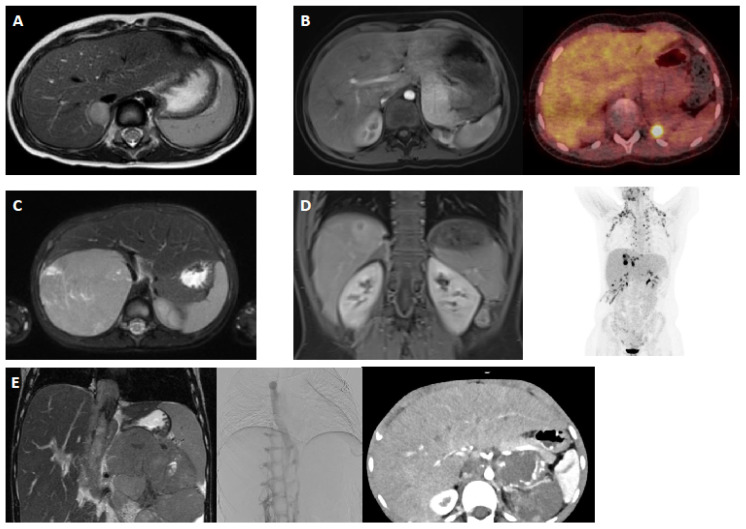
Image examples of the five patient cases: (A) An axial T2w image of the right-sided adrenocortical carcinoma of patient 1. (B) An axial T1w image with fat saturation post contrast and axial F18-FDG-PET/CT fusion of the left-sided adrenocortical carcinoma of patient 2. (C) An axial T2w image with fat saturation of the right-sided adrenocortical carcinoma of patient 3. (D) A coronal T1w image post contrast and whole-body MIP of the right-sided adrenocortical carcinoma of patient 4. (E) A coronal T2w image, cavography (with obstruction of VCI) and axial CT scan post contrast of the left-sided adrenocortical carcinoma of patient 5.
